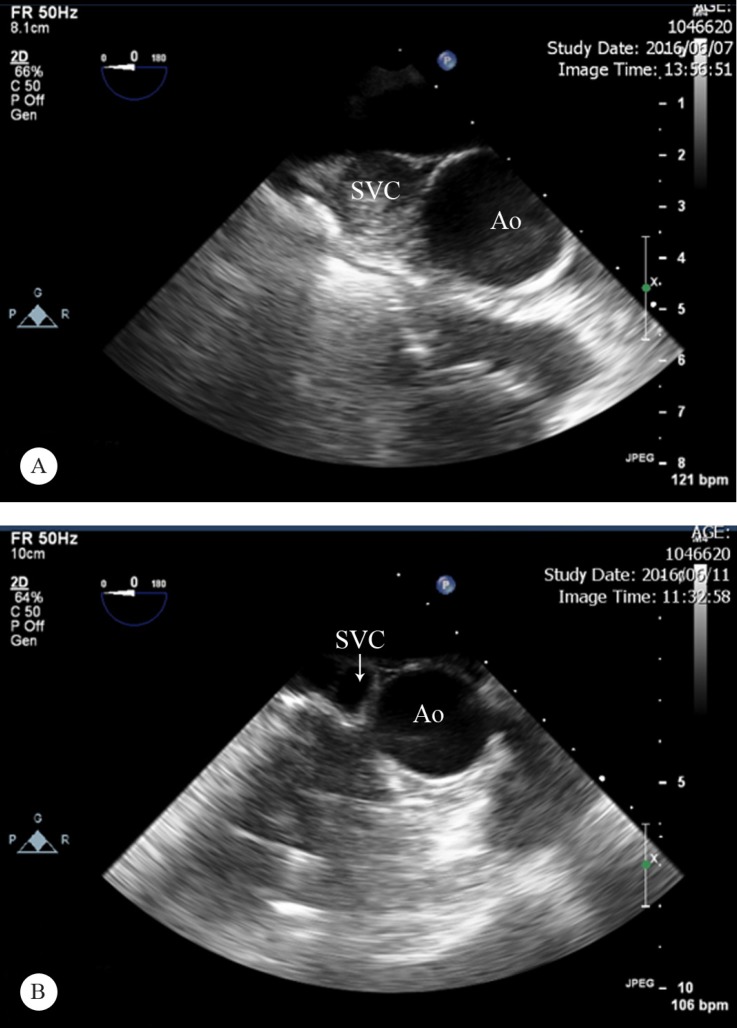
Transesophageal echocardiography in the upper esophageal view before catheter-directed thrombolysis (A), showing a dilated (20 mm) SVC totally obstructed by thrombus, and then a patent SVC (arrow) with complete resolution of thrombosis after treatment (B).
